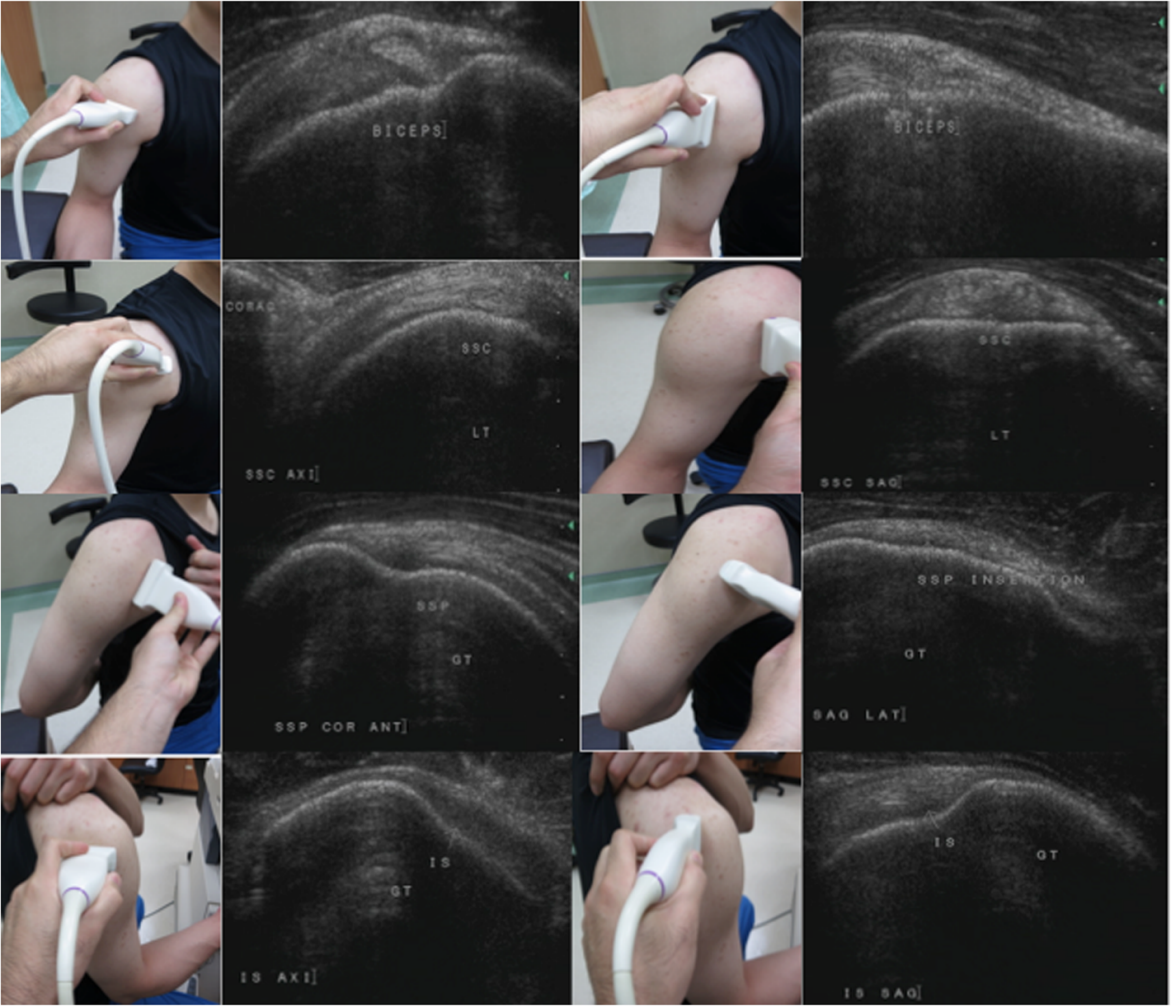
Positions in shoulder ultrasonography examination
